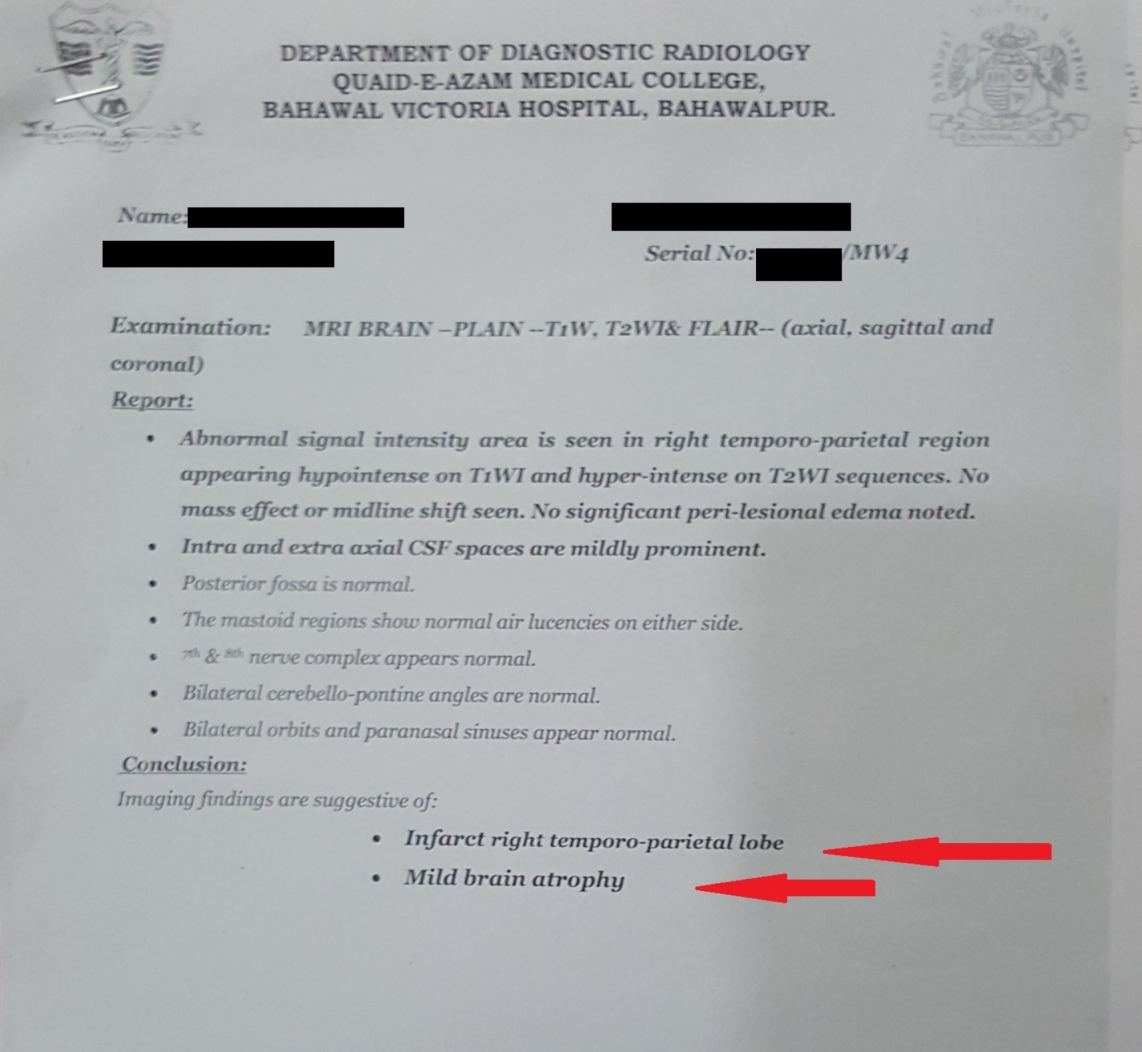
Magnetic resonance imaging report
